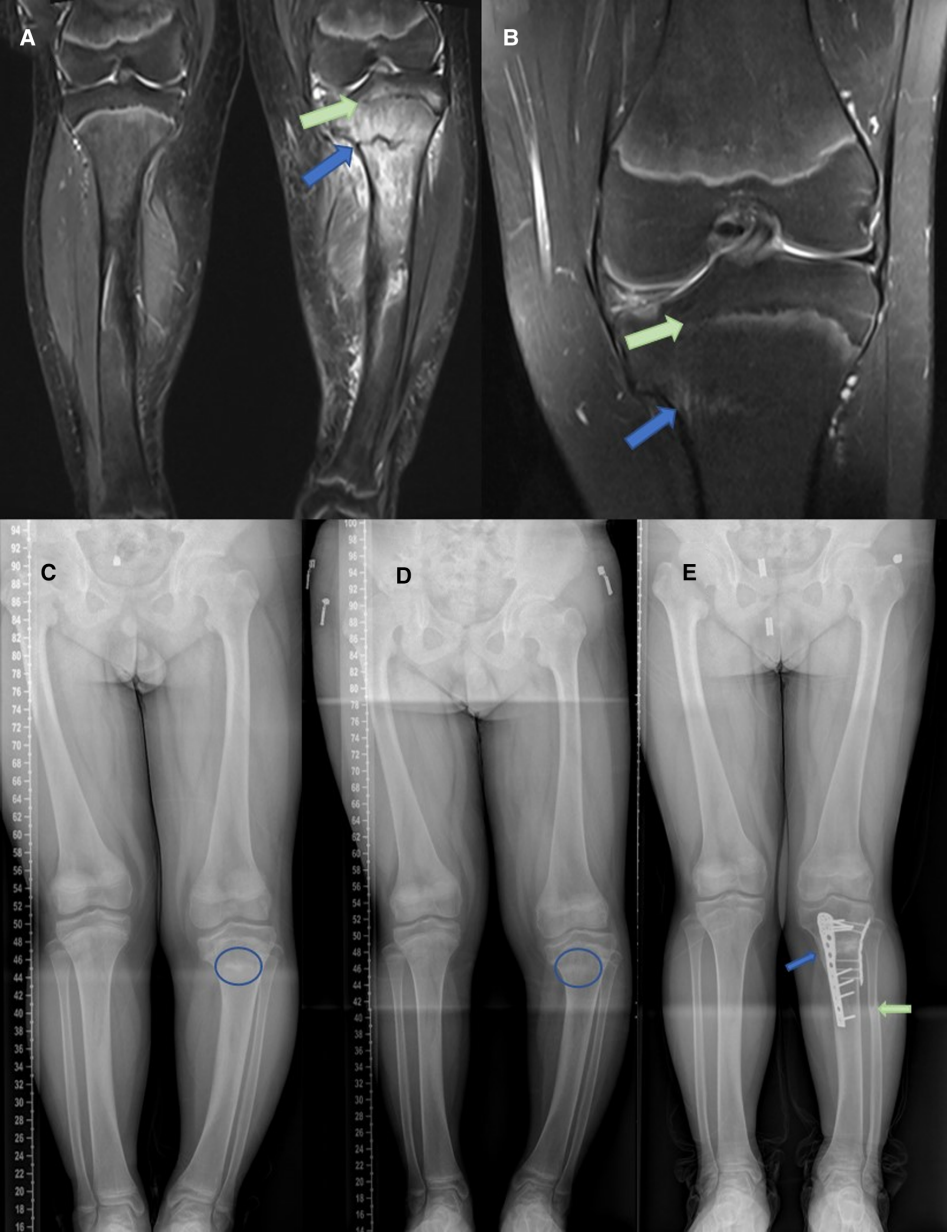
(A) T2-weighted MRI demonstrated a rough, high signal fracture line, surrounded by extensive high signal bone marrow edema (blue arrow) with a compression injury to the medial tibial growth plate (Salter V fracture) (green arrow). (B) After 3 months of conservative treatment, the stress fracture line disappeared and the Salter V fracture was clearly observed (C–E). Changes of the patient's lower limb force line. The blue circle represents the range of stress fractures. The arrow represents the location of the osteotomy.
